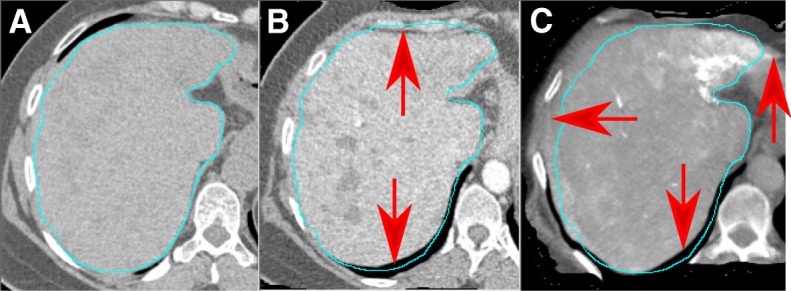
An example (patient ID BI02 from training set in our database) of mis-registration for CT_FDG_ and CBCT to CT_MAA_ non-rigid registration (Likert score for this patient is “little misalignment,” contours come from automatic liver segmentation and red arrows point to mis-registration area. a Reference image (CT_MAA_), b deformed CT_FDG_, c deformed meta-CBCTs
